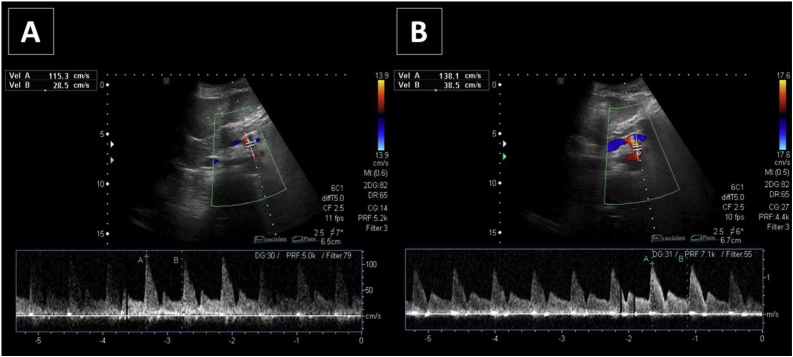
Postoperative spectral Doppler US with patient in supine decubitus, during inspiration (4A) and expiration (4B), showing the change in the peak systolic velocity during expiration after surgery, that became normal (138.1 cm/s).
